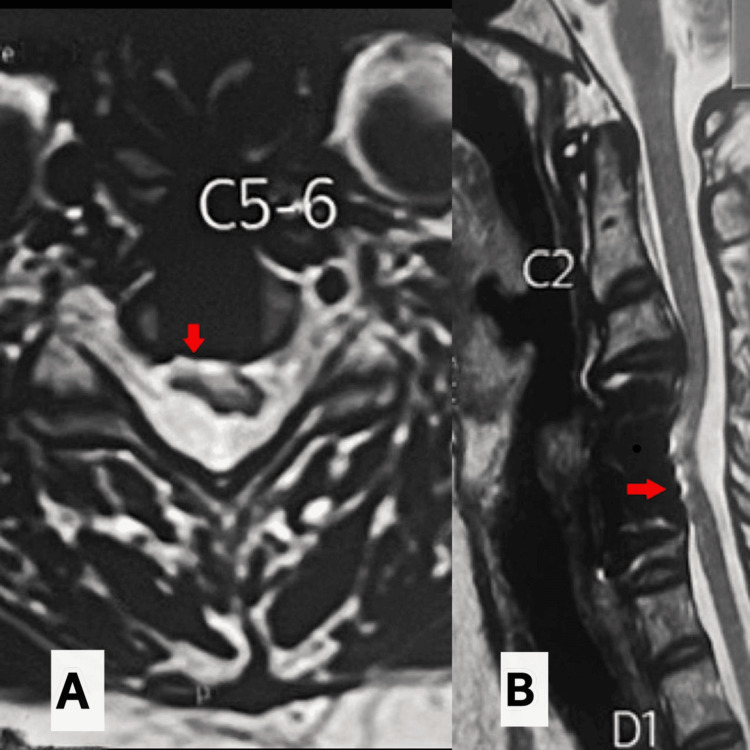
Two-year follow-up MRI demonstrating restoration of the ventral cerebrospinal fluid (CSF) column. (A) Axial image at the C5-6 level shows re-established ventral CSF space with residual cord thinning (arrow). (B) Sagittal image demonstrates restoration of the ventral CSF column at the level of previous ventral displacement, with associated focal myelomalacia and relative widening of the dorsal CSF space (arrow), consistent with chronic remodeling. MRI: magnetic resonance imaging, C5-6: cervical 5-6
